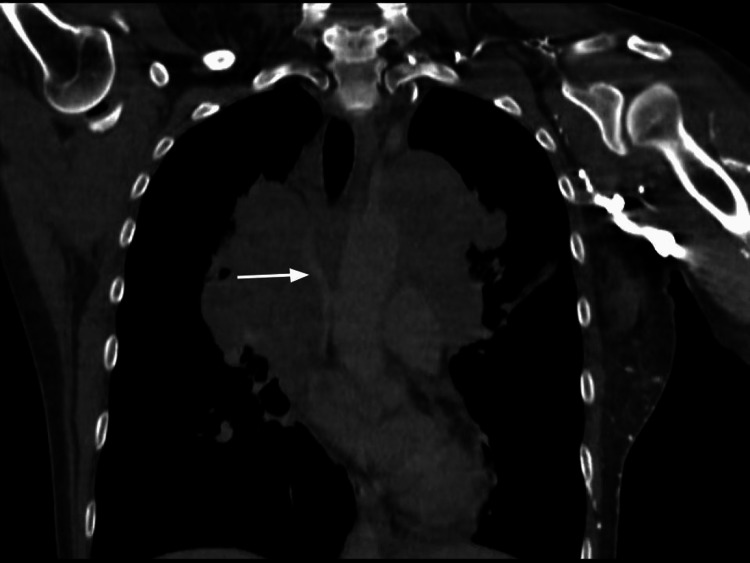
CT scan of the chest with contrast (sagittal view) showed marked narrowing of the mid-to lower-level superior vena cava (white arrow).
